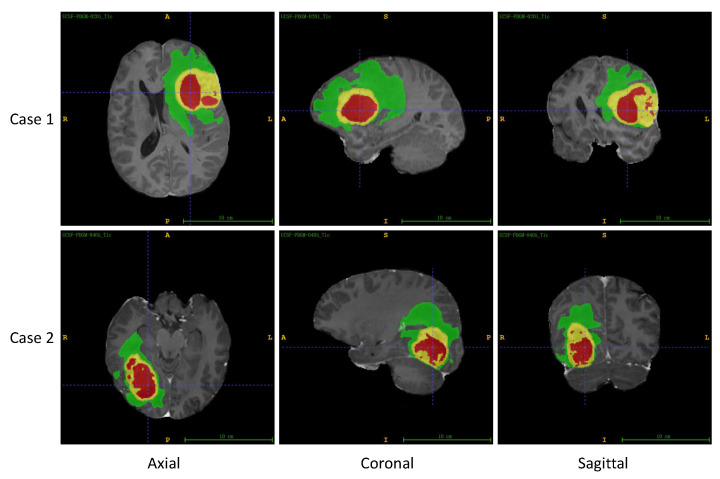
Two sets of MRI images and corresponding segmentation labels from UCSF-PDGM dataset, including axial, coronal, and sagittal views. In this figure, ET is labeled yellow, TC is the union of yellow and red, and WT includes all the colored (yellow, red, and green) labels.
